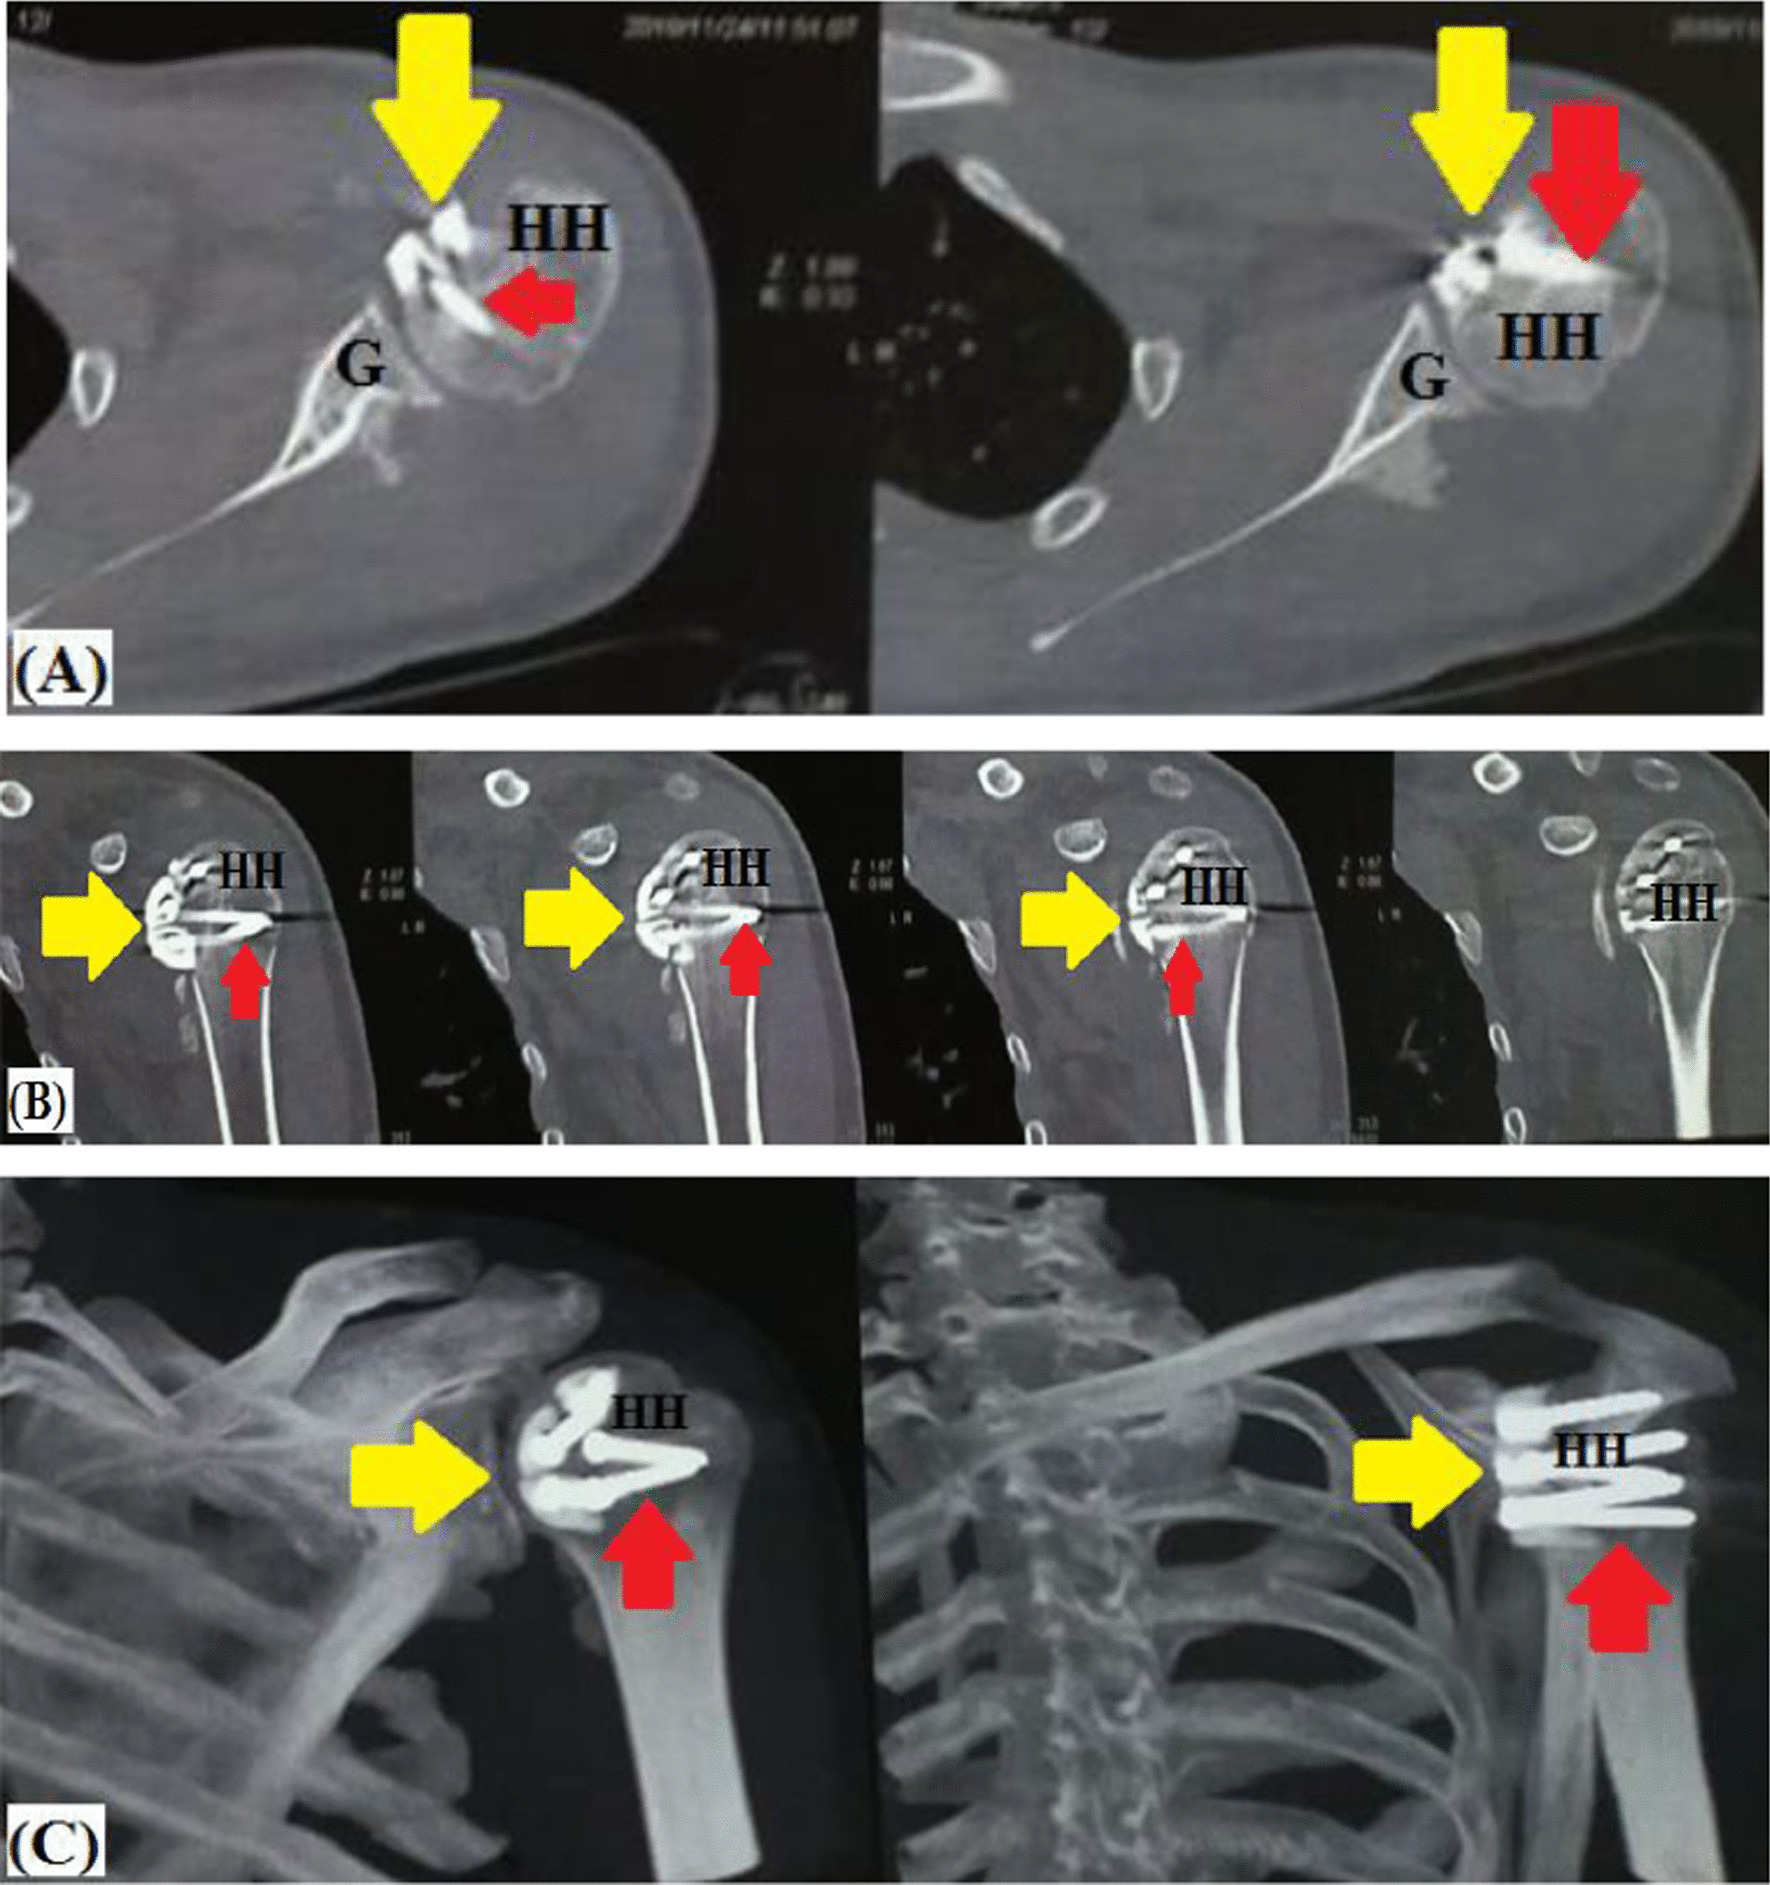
Postoperative imaging of restored humeral head sphericity and articular congruity following (red arrow-marked) screw fixation of (yellow arrow-marked) fibular graft pieces into reverse Hill-Sachs defect in left shoulder; A axial CT image; B coronal CT image; C 3D-reconstruction CT image; G, Glenoid; HH, Humeral head
